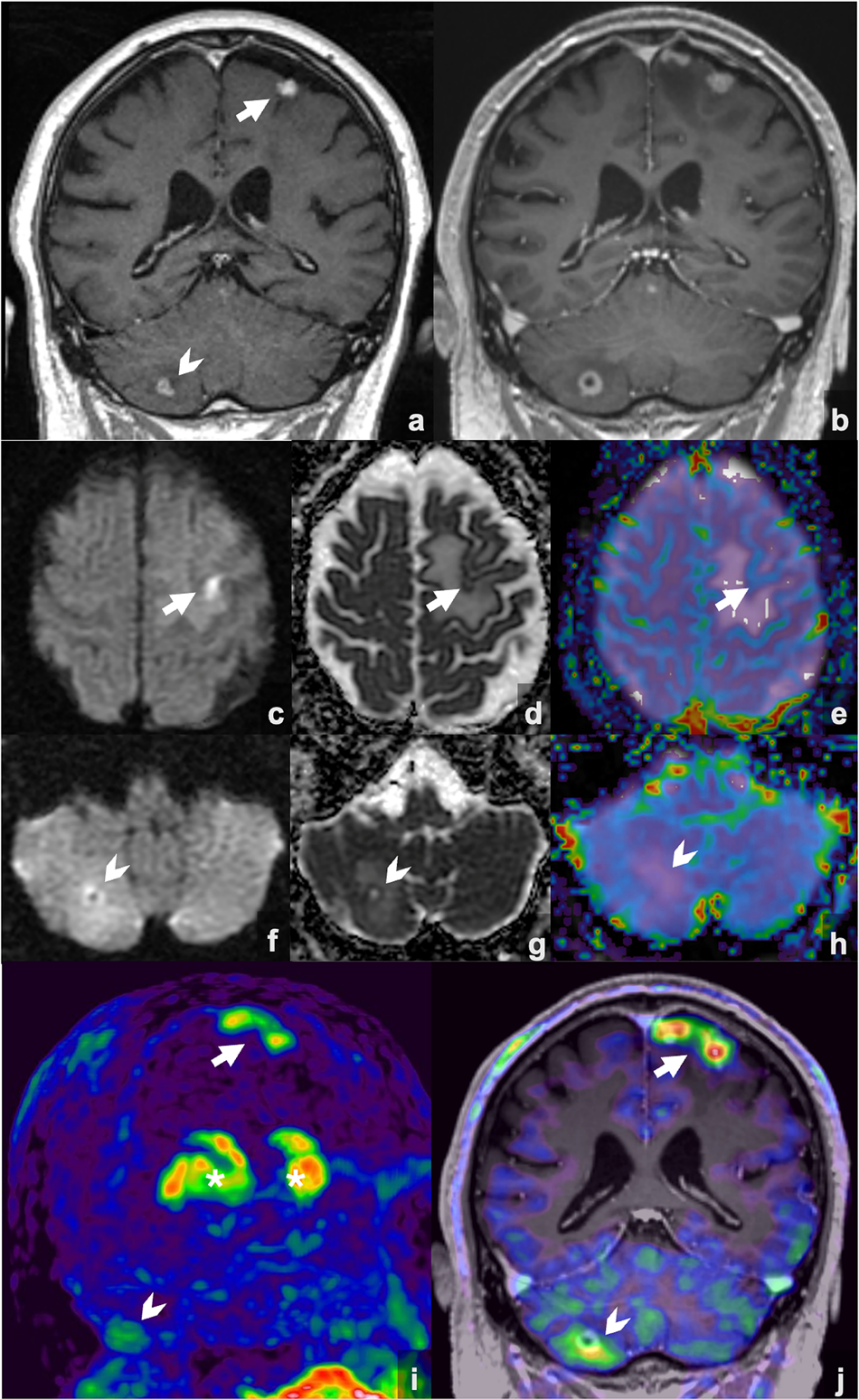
Brain MRI and ^18^F-FDOPA PET in NSCLC brain metastases after Pembrolizumab and SRS. A left frontal cortico-subcortical lesion (arrow) and a right cerebellar hemisphere lesion (arrowhead) are shown (a). One year post-treatment, MRI (b) shows enlargement of both lesions and a new nodularity adjacent to the frontal lesion. Diffusion and perfusion imaging were inconclusive for the frontal lesion (DWI c, ADC d, rCBV e). The cerebellar lesion showed peripheral increased signal on the DWI (f) with decreased ADC (g) but no rCBV increase (h). ^18^F-FDOPA PET (3D reconstruction i, fused MRI-PET j) demonstrated two foci of uptake in the frontal lesion (arrow), consistent with active disease, while the cerebellar lesion showed only mild uptake (arrowhead), suggesting post-treatment changes. Physiologic ^18^F-FDOPA uptake is noted in the basal ganglia (*)
